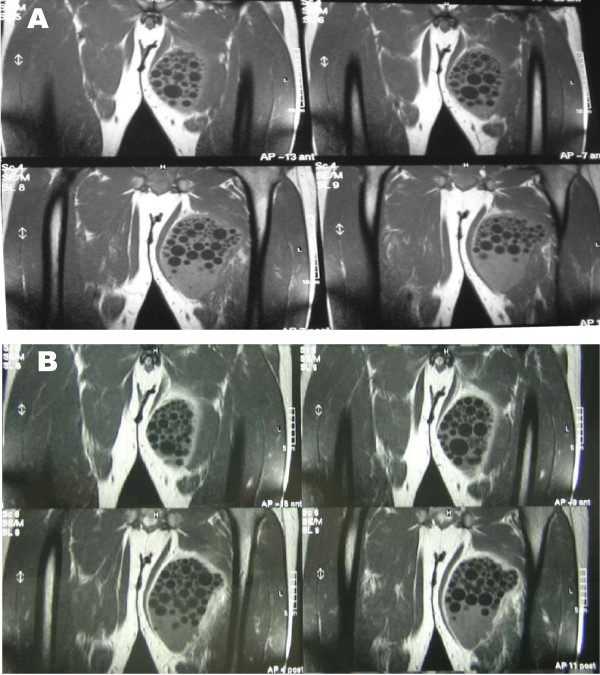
MRI of the left thigh, sequence T1-weighted: a) precontrast image: "ovular mass in the adductor magnus muscle, with a cystic aspect, dimensions 13 × 8 cm containing numerous cystic formations with regular outlines; b) after contrast image: "the lesion appears surrounded by a thin wall homogeneously impregnated after the injection of paramagnetic contrast medium while there is no contrastographic enhancement of the content of the lesion".
